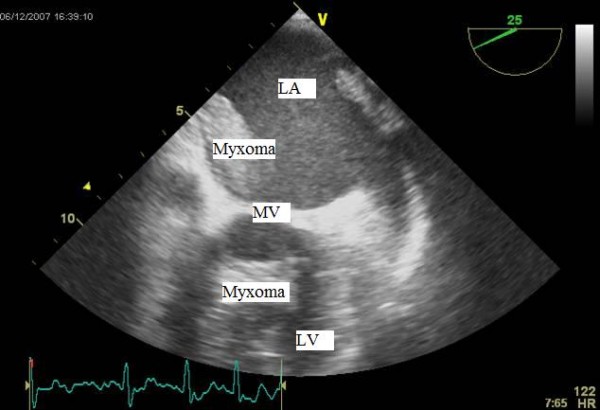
Trans-oesophageal echocardiographic (TOE) image of the left atrial myxoma obstructing the mitral valve. The two chamber view shows the tumor prolapsing through & obstructing the mitral valve in diastole (LA: left atrium, LV: left ventricle).
